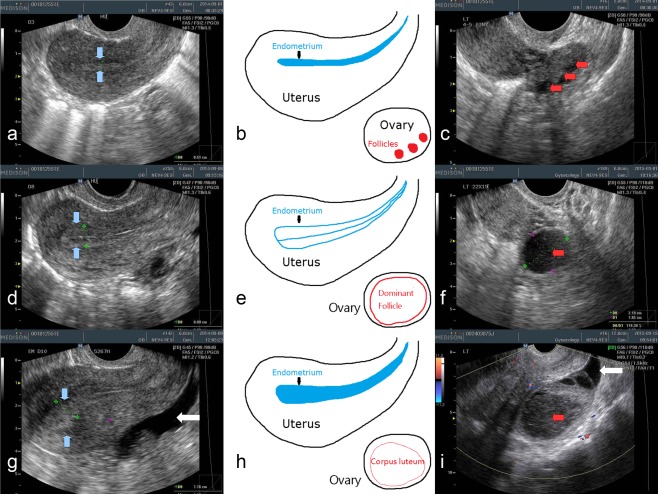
Transvaginal ultrasonography of ovary and endometrium in early follicular phase (a–c), late follicular (shortly before ovulation) phase (d–f), and post‐ovulatory luteal phase (g–i). In early follicular phase, the endometrium (between the blue arrows) just after menstruation appeared thin and homogenous (a). Multiple small follicles (red arrows) could be seen inside the ovary (c). In late follicular phase, the endometrium was thickened, and a typical “triple line” appearance could be seen (d). A dominant follicle, approximately 2 cm in diameter, is about to ovulate in late follicular phase (f). After ovulation, the endometrium becomes “luteinized,” with increased echogenicity (whitening) (g). The dominant follicle transforms into a corpus luteum (i). Note the increased echogenicity and inhomogenous content (with filament‐like structure) inside the corpus luteum compared to the dominant follicle in picture f. Free fluid can be seen in the pelvis (white arrows in pictures g and f)
